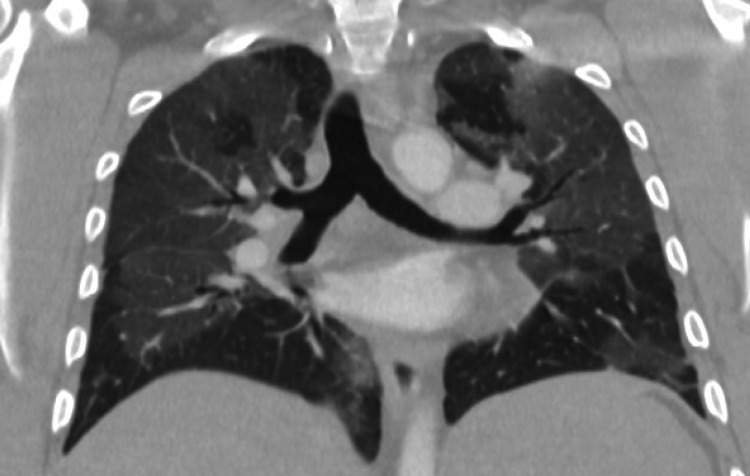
Coronal CT in lung windows shows diffuse ground-glass opacities bilaterally. Findings are overall nonspecific but can be seen with viral/atypical pneumonia.
